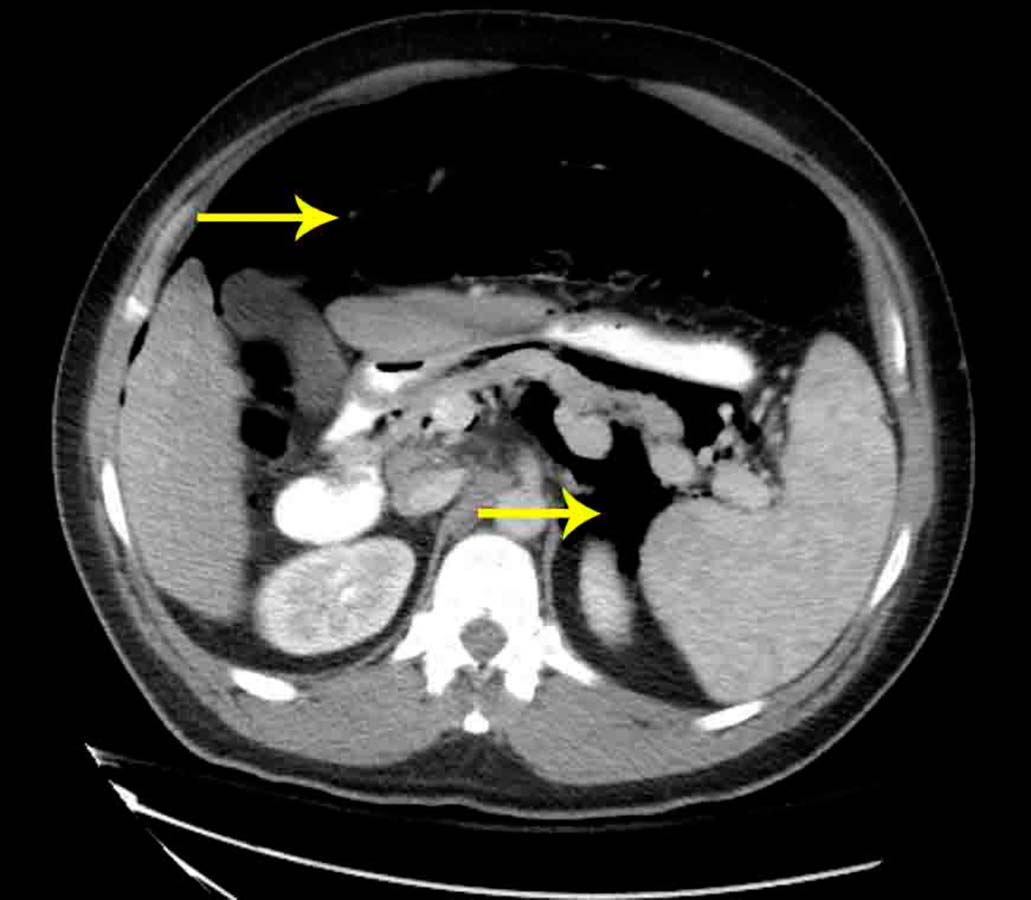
CT showing pneumoperitoneum (top arrow) and pneumoretroperitoneum (bottom arrow) following cryoablation. CT, computed tomography.
